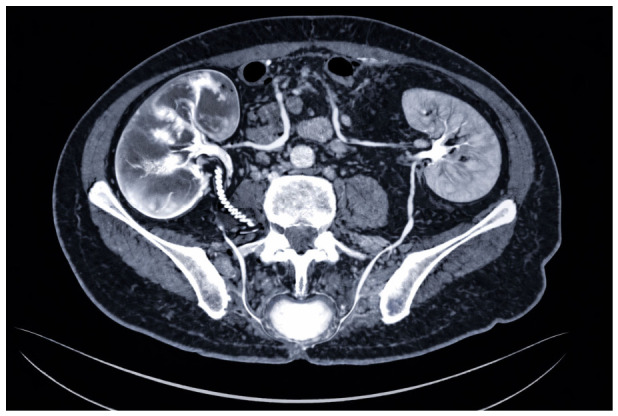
Contrast-enhanced CT scan showing the dislocated, migrated right ureteral stent.
